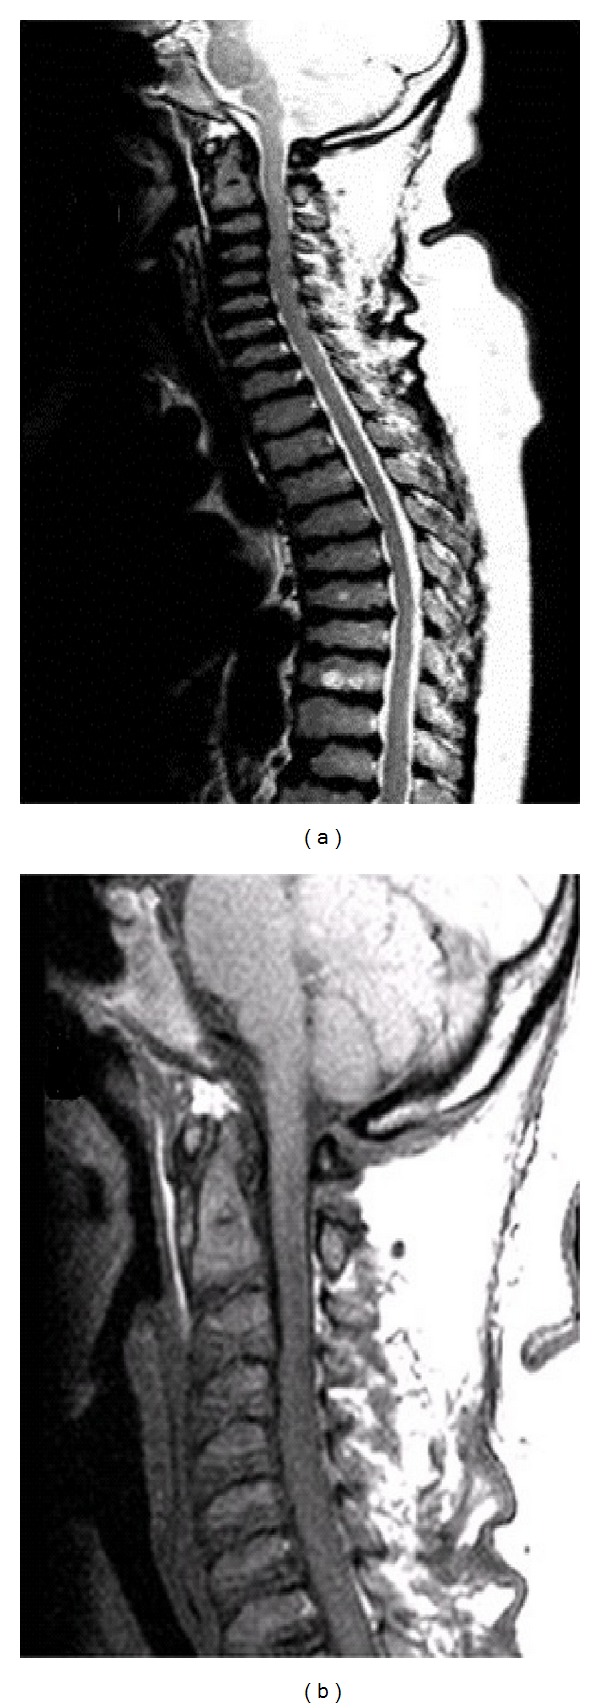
(a) MRI sagittal TSE T2 view of the cervico-thoracic spine. (b) MRI sagittal T1 view of the cervical spine showing hypoplasia of the odontoid process with a mild cord compression at the level of C2-C3.
